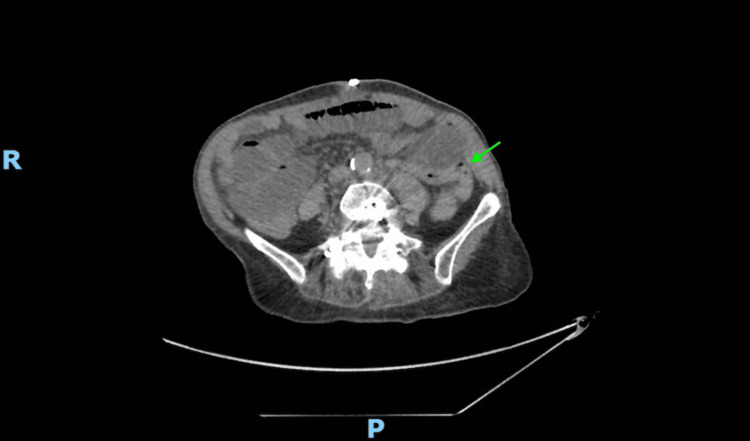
Axial view of CT of the abdomen showing dilated small bowel loops with angulation of the bowel loops in the left iliac fossa (green arrow)
